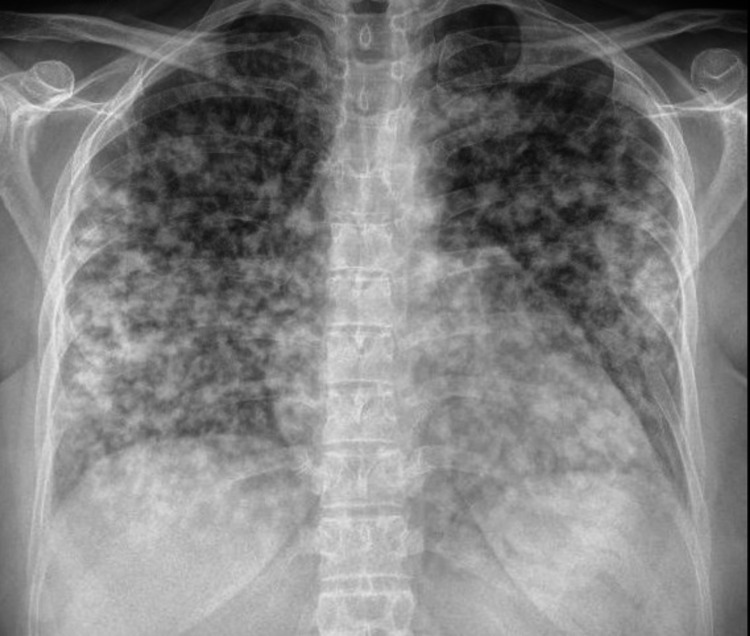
Chest X-ray images revealed bilateral patchy infiltration.
